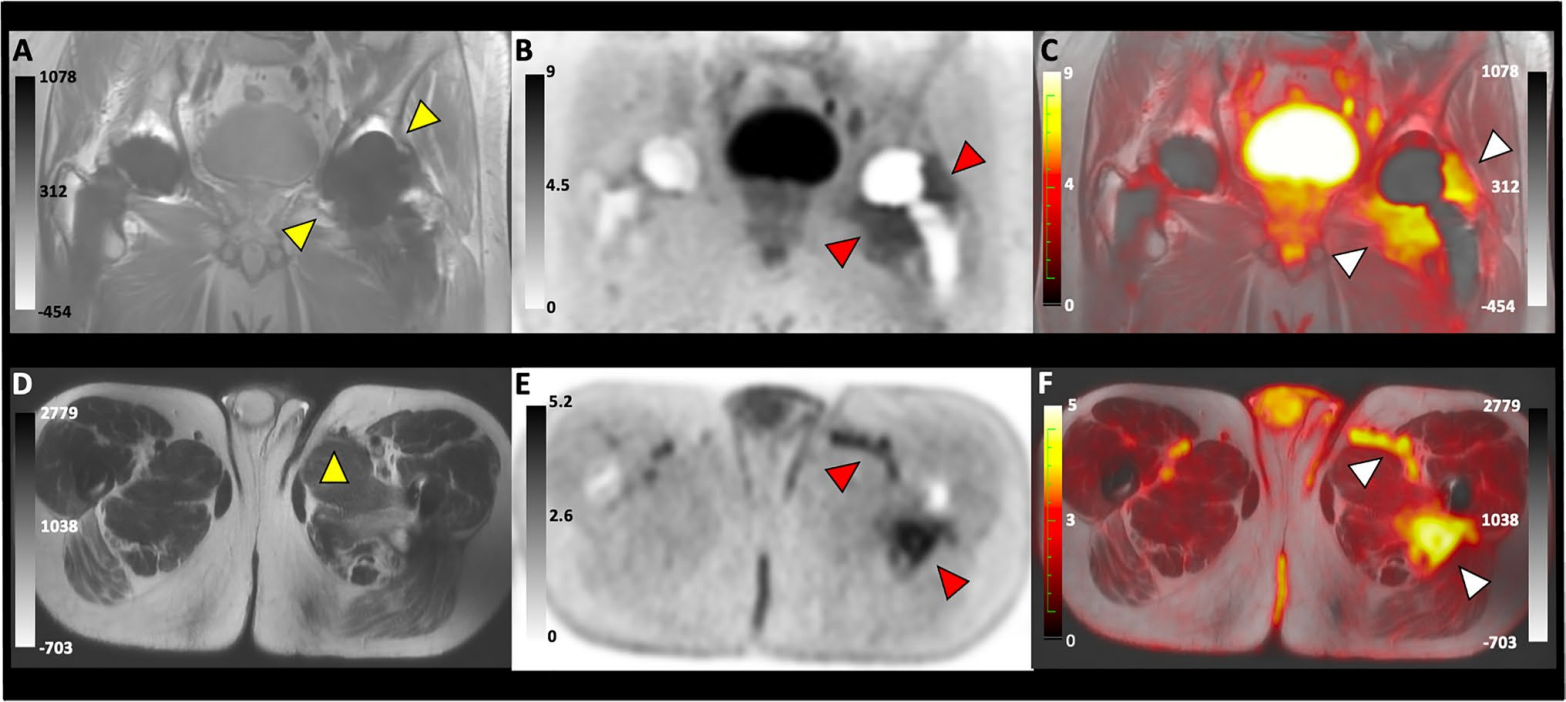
Images for D-^11^C-Met PET/MRI of a 61-year-old male with bilateral hip prosthesis and confirmed PJI of the left hip. (A)–(C) Show coronal images of MRI, PET, and Fused PET/MRI scan, respectively. The yellow arrows in (A) point to the infected joint. Red and white arrows in (B) and (C) point to at the area of D-^11^C-Met uptake surrounding the joint in both the PET and fused images, respectively. (D)–(F) show axial images of MRI, PET, and Fused PET/MRI scan, respectively. The yellow arrow in (D) points at the sinus tract in the abductor muscles. Red and white arrows in (E) and (F) point at the area of D-^11^C-Met uptake in the sinus tract in both the PET and fused images, respectively
